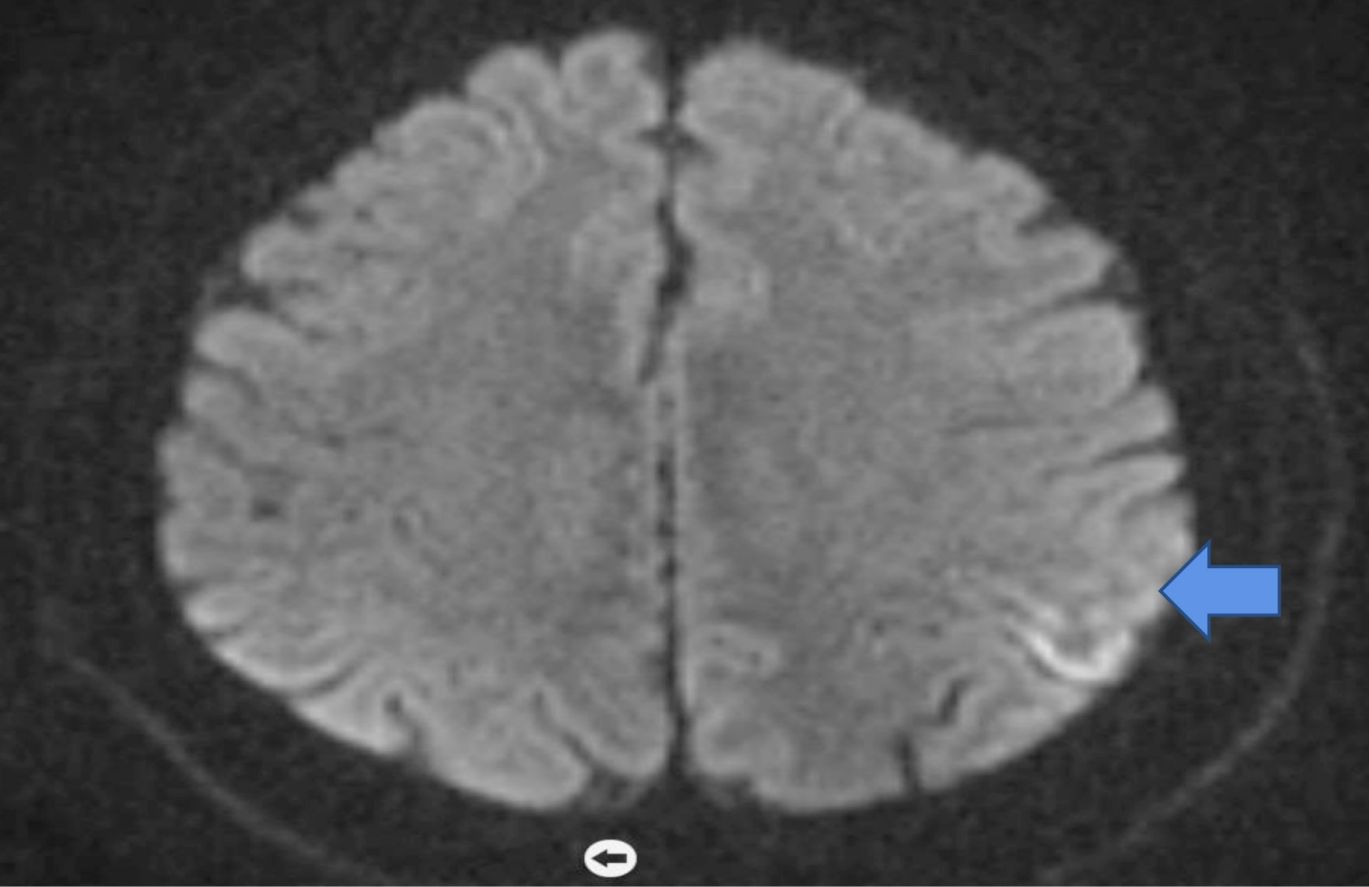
Diffusion-weighted magnetic resonance imaging of the brain
